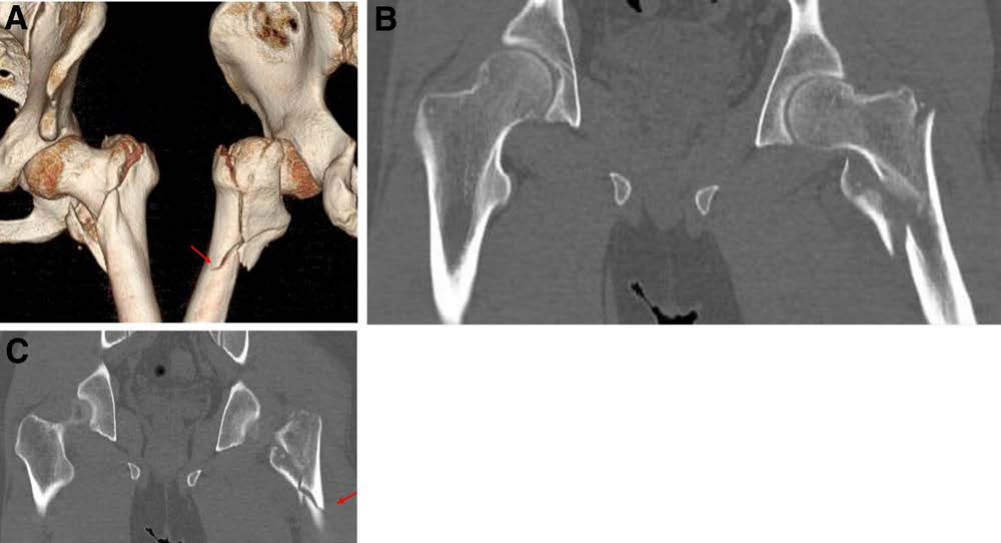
Computed tomography (CT) images showing (A) a 3D reconstruction and (B and C) coronal views of an intertrochanteric femoral fracture (AO/OTA 31-A3) in a 38-year-old patient (red arrow).
